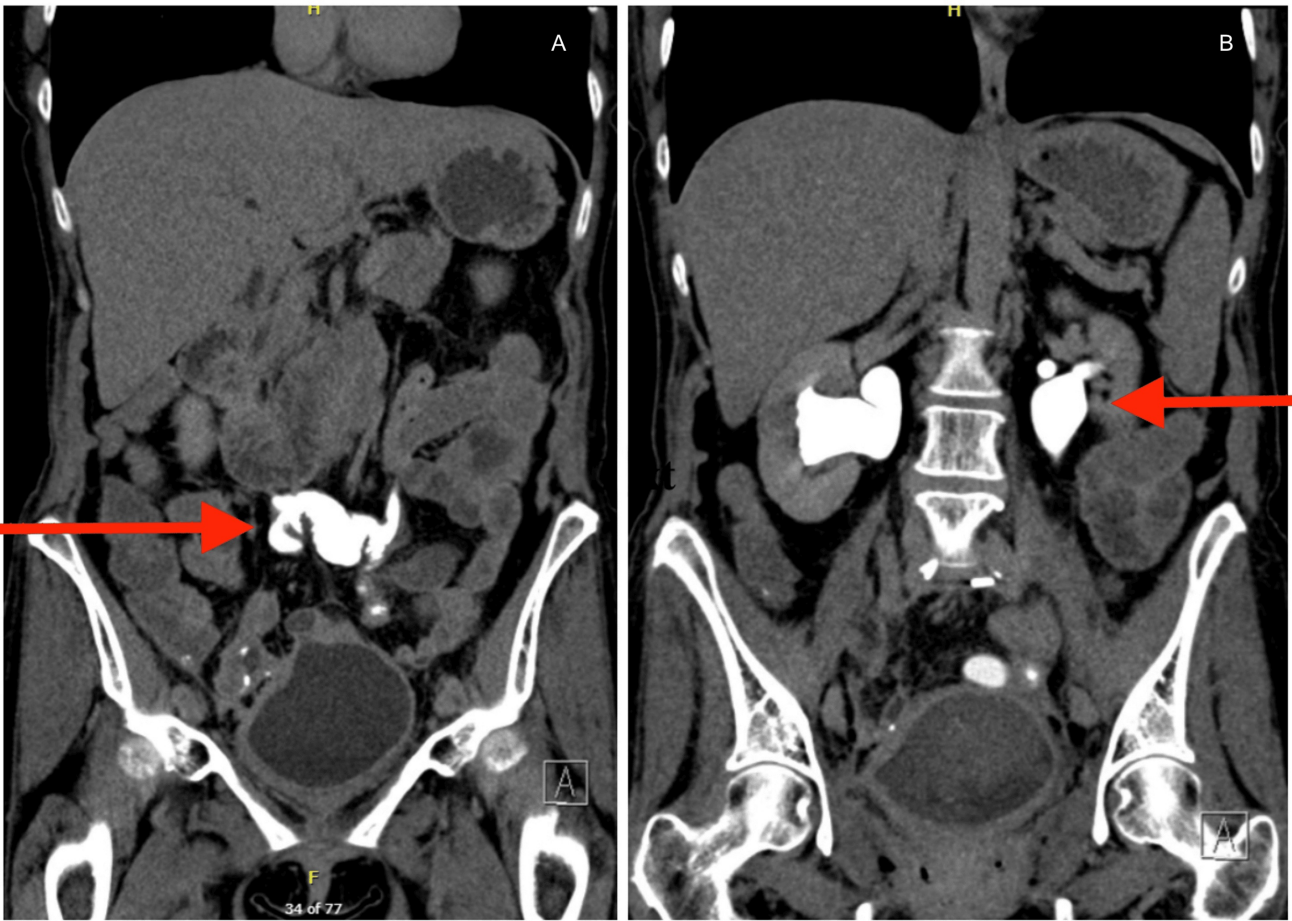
CT urogram a: coronal CT image demonstrating the urinary drainage site (red arrow) following distal ureteral resection with creation of a single ileal conduit connecting the bilateral ureters to the bladder dome; b: coronal CT image demonstrating bilateral hydroureteronephrosis (red arrow) status post double-J nephroureteral stent removal, likely secondary to vesicoureteral reflux. Postsurgical changes include prior abdominopelvic resection with left lower quadrant ileostomy and coloanal anastomosis.
